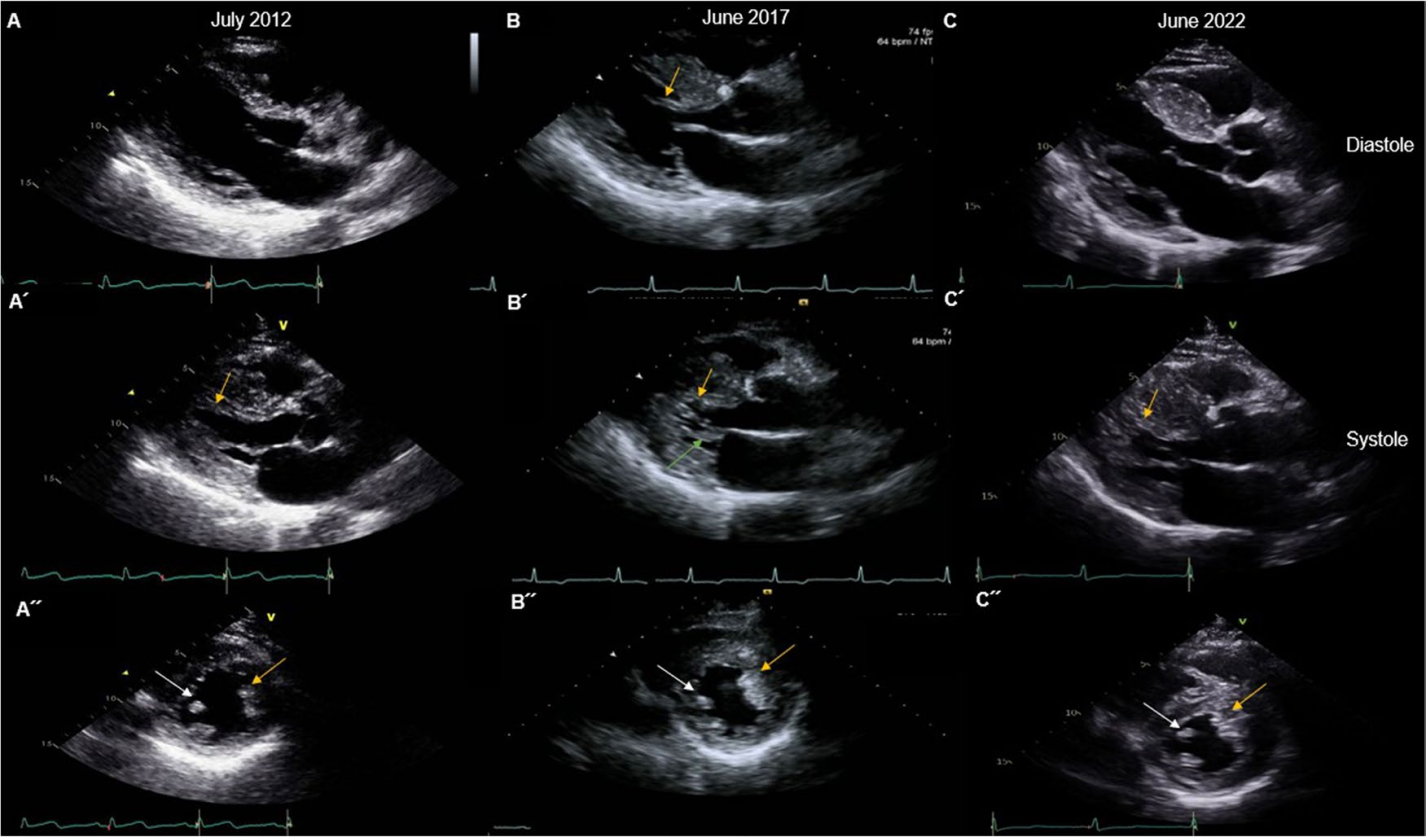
Serial echocardiography at baseline, 5-year, and 10-year follow-up. Echocardiography revealed non-obstructive hypertrophic cardiomyopathy with left ventricular hypertrophy that had gradually progressed over 10 years. The left (A), middle (B), and right (C) panels indicate echocardiographic images at baseline (July 2012), 5-year follow-up (June 2017), and 10-year follow-up (June 2022), respectively. The upper and middle panels are parasternal long-axis echocardiographic images acquired at diastole and systole. Lower panels are images for the parasternal short axis at the papillary muscle level
